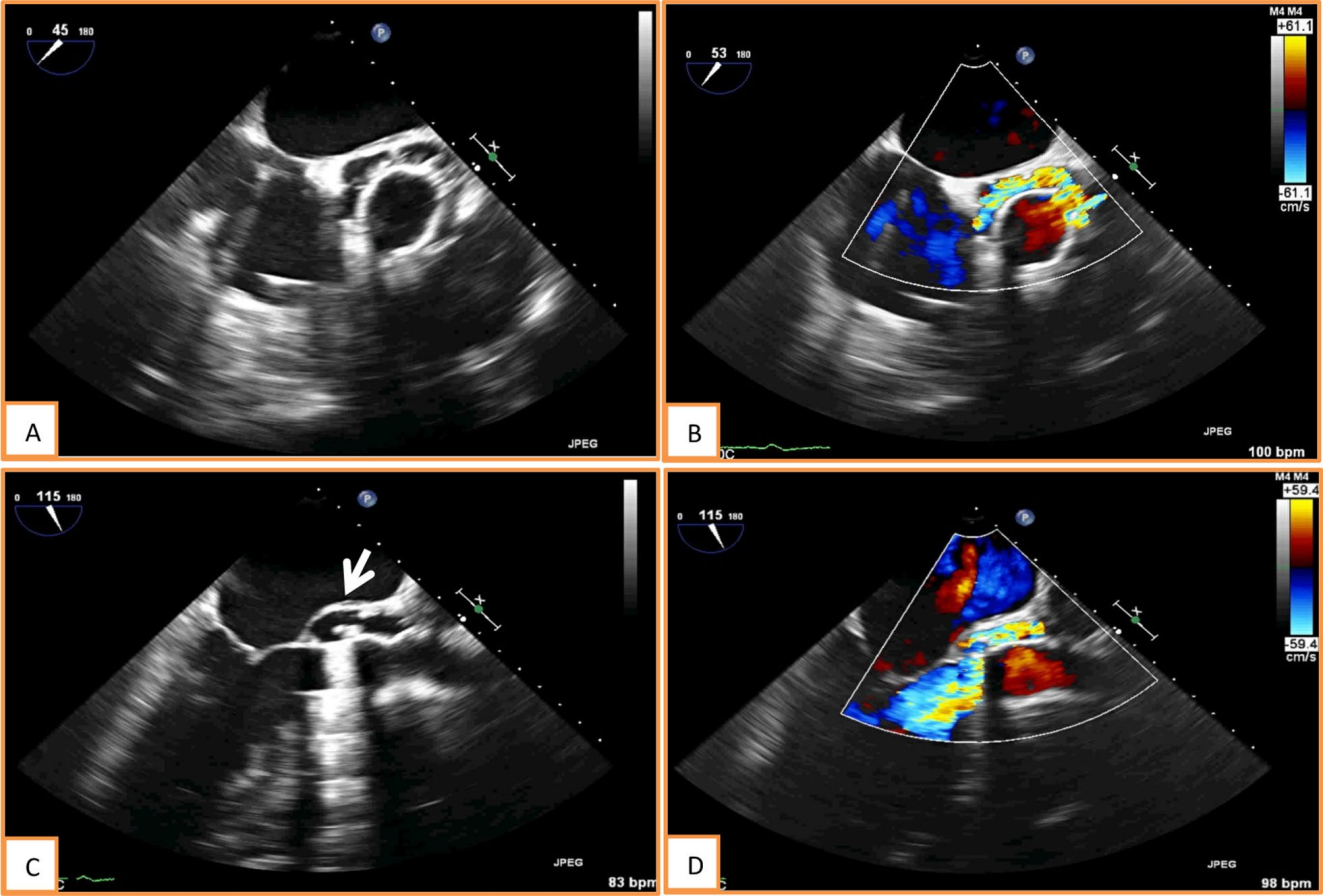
Transesophageal echo A short-axis (SA) view showing dehiscence (arrow); B SA color Doppler; C pseudoaneurysm in 3-chamber view (arrow); D 3-chamber color Doppler
